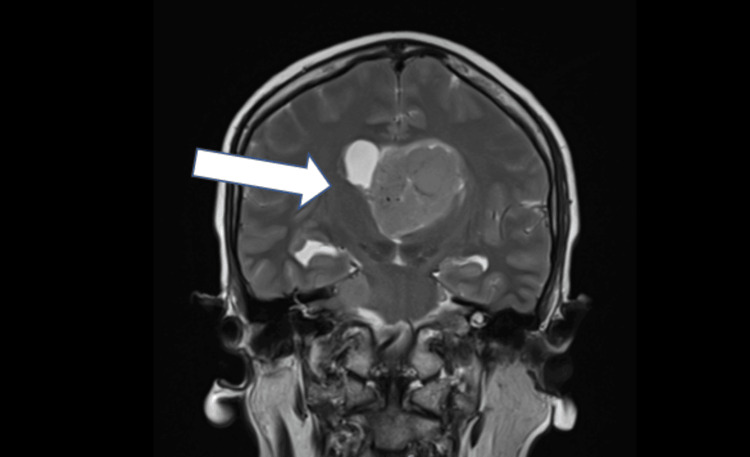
A coronal view of brain MRI showing a centrally located lesion within the third ventricle (white arrow) with a lobulated morphology and significant distortion of surrounding structures, including the compression of adjacent brain tissue and effacement of nearby cortical sulci MRI: magnetic resonance imaging
